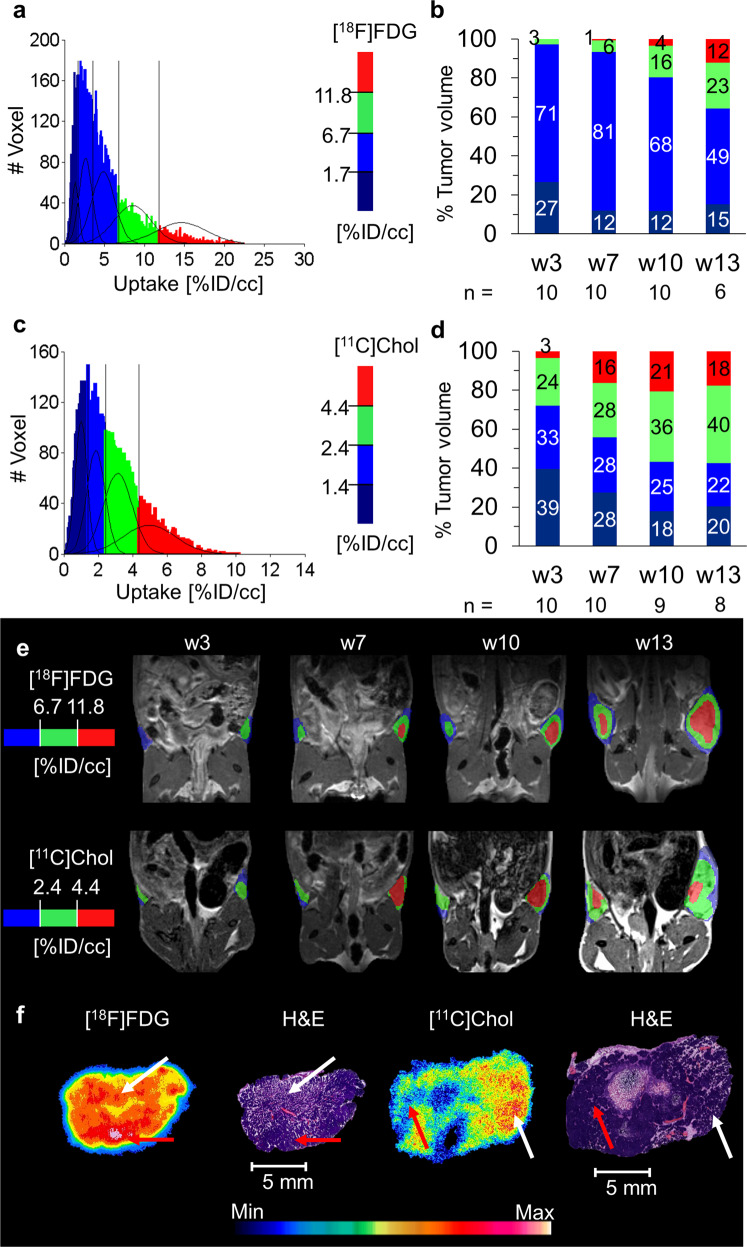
[^18^F]FDG and [^11^C]Chol clustering mismatch identifies tumor stages. a The summed and fitted histogram of [^18^F]FDG, as well as b the volumetric percentage of the uptake populations within the lesions for every time point, demonstrated a high amount of the low [^18^F]FDG uptake populations within the lesions in this study, including at later time points. The high uptake population (> 11.8%ID/cc) showed a slight increase over time up to 12% of the late-stage lesions in w13. c The summed histogram of all time points of [^11^C]Chol was analyzed as described for [^18^F]FDG, and the sum of four Gaussian distributions were identified as the best model. d The volumetric percentage of the uptake populations within the lesions for every time point demonstrated a lower amount of low [^11^C]Chol uptake populations compared to [^18^F]FDG. After a slight increase of the higher uptake populations (green, 2.4–4.4%ID/cc and red, > 4.4%ID/cc) from w3 to w7 and w10, both remained relatively stable until w13. e The parametric maps showed the appearance of the highest uptake populations at w7 in single tumors for both tracers, staying stable over time for [^11^C]Chol and increasing for [^18^F]FDG. f The autoradiography results of representative tumor slides demonstrated the highest [^18^F]FDG uptake in IC regions (red arrows), whereas the highest [^11^C]Chol uptake was observed in proliferating regions including DCIS-like high-grade MIN (white arrows) rather than invasive tumor regions.
